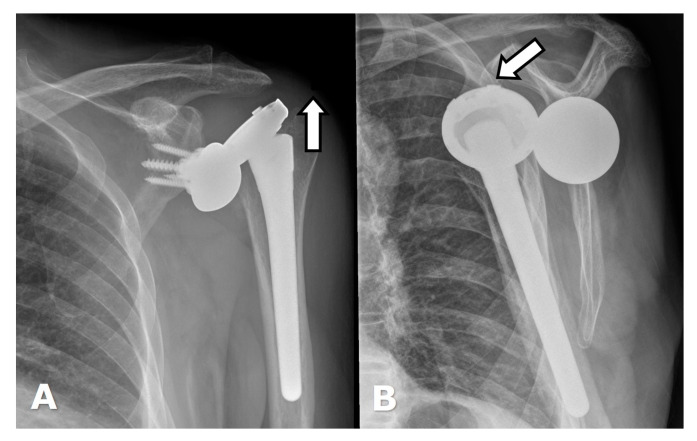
Dislocated RTSA. (A). Frontal radiograph shows the humeral component displaced superiorly (arrow denotes force direction). (B). Scapular Y view shows the humeral component displaced anteriorly (arrow denotes force direction).
